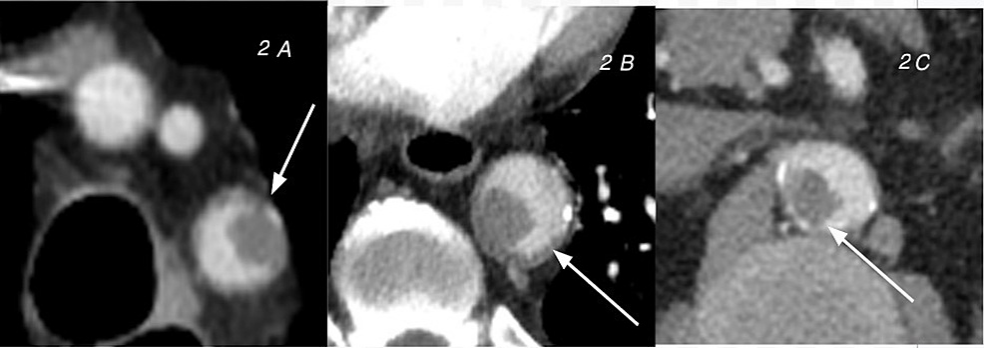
Case 2. CT angiography per the pulmonary embolism protocol shows free-floating thrombosis in the arch (A), descending aorta (B), and abdominal aorta (C). CT, Computed tomography
